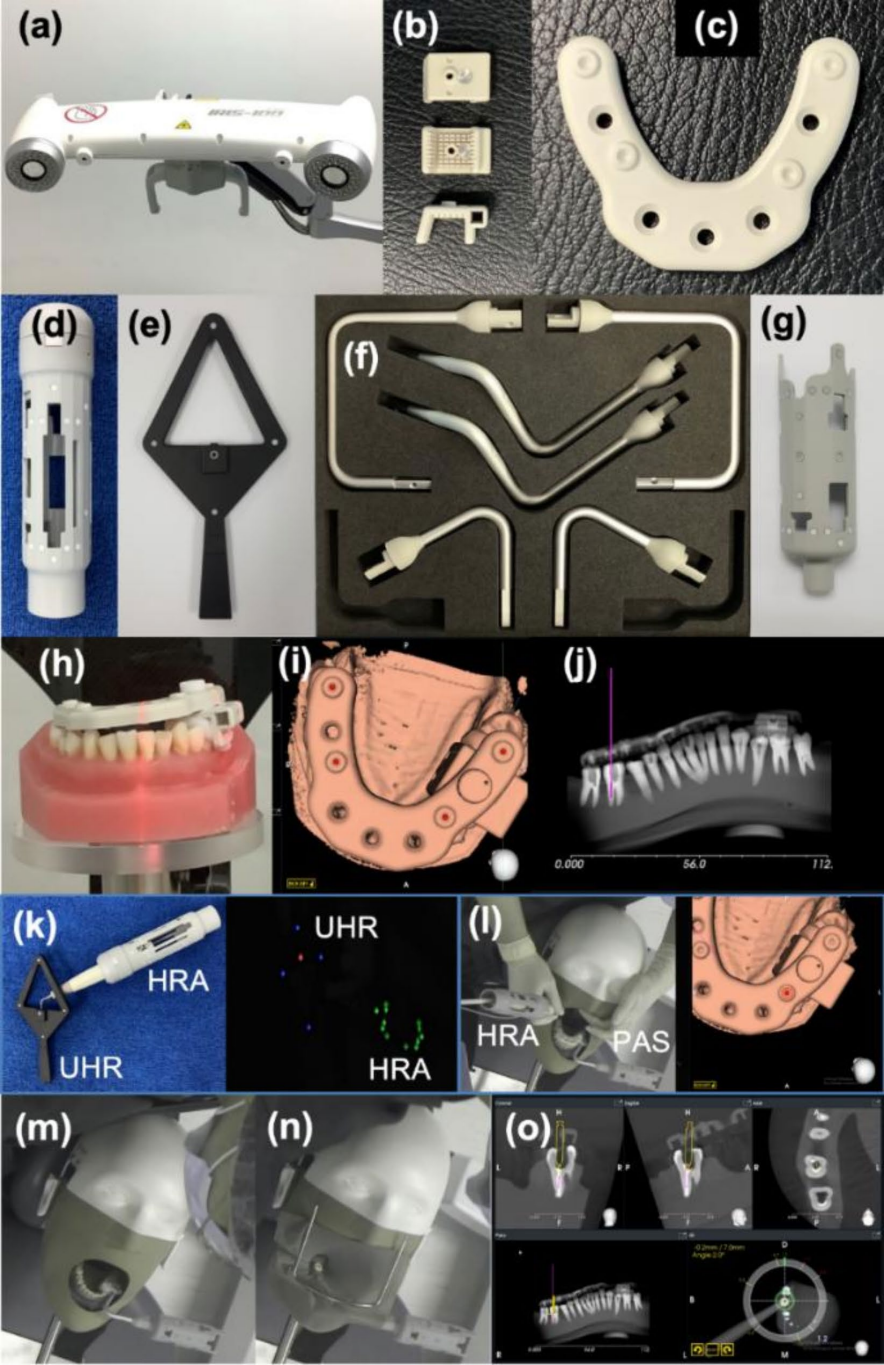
The instruments used in DNS system and digital workflow. Pre-experiment photographs in Fig. 2a − 2h captured by a digital camera. Intra-experiment photographs in Fig. 2i − 2o captured from IRIS-100 software. The instruments used in the DNS system were (a) Tracking unit (TU), (b) Holding tray (HT), (c) Occlusal guide appliance with four radiopaque fiducial markers (OGA), (d) Handpiece reflective attachment (HRA), (e) Ultrasonic handpiece registrator (UHR), (f) Jaw attachment (JA), and (g) Positioner arm set (PAS). (h) CBCT radiographic examination in mandibular casts-HT-OGA, (i) Feature point registration planned, (j) Added fiber post in the DNS plan, (k) Instrument registration, (l) Feature point registration, (m) HT-JA-PAS was attached to the cast, (n) Rubber dam was placed on the cast, and (o) Fiber post removal using guidance mode in the DNS system
